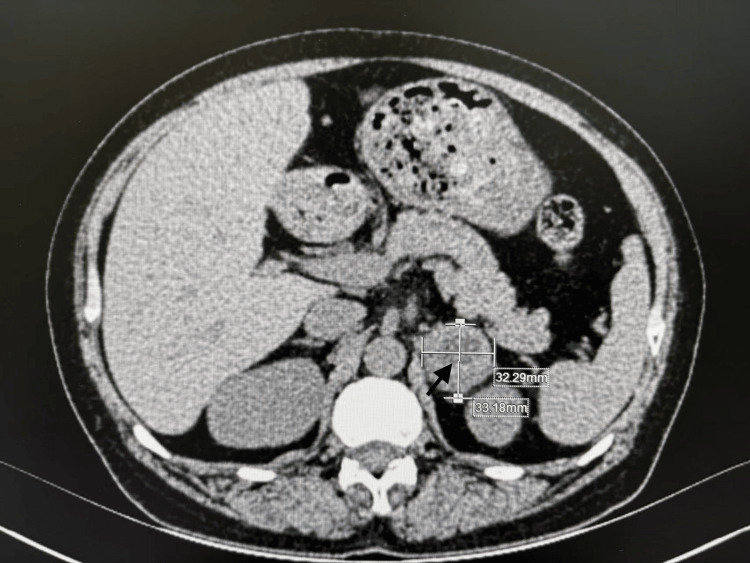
Abdominal CT scan showing a mixed-attenuation left adrenal mass (indicated by the arrow), measuring approximately 3.3 × 3.2 cm CT: computed tomography
